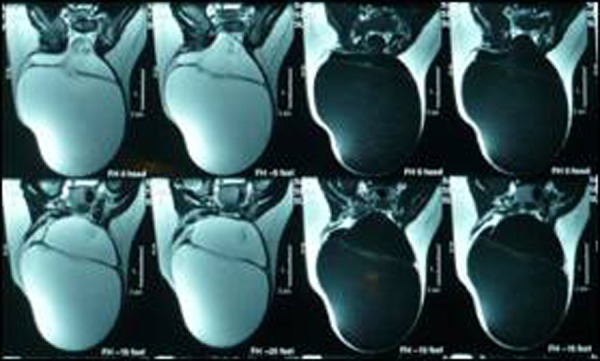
Figure 3: MRI in coronal section showing double compartment swelling
